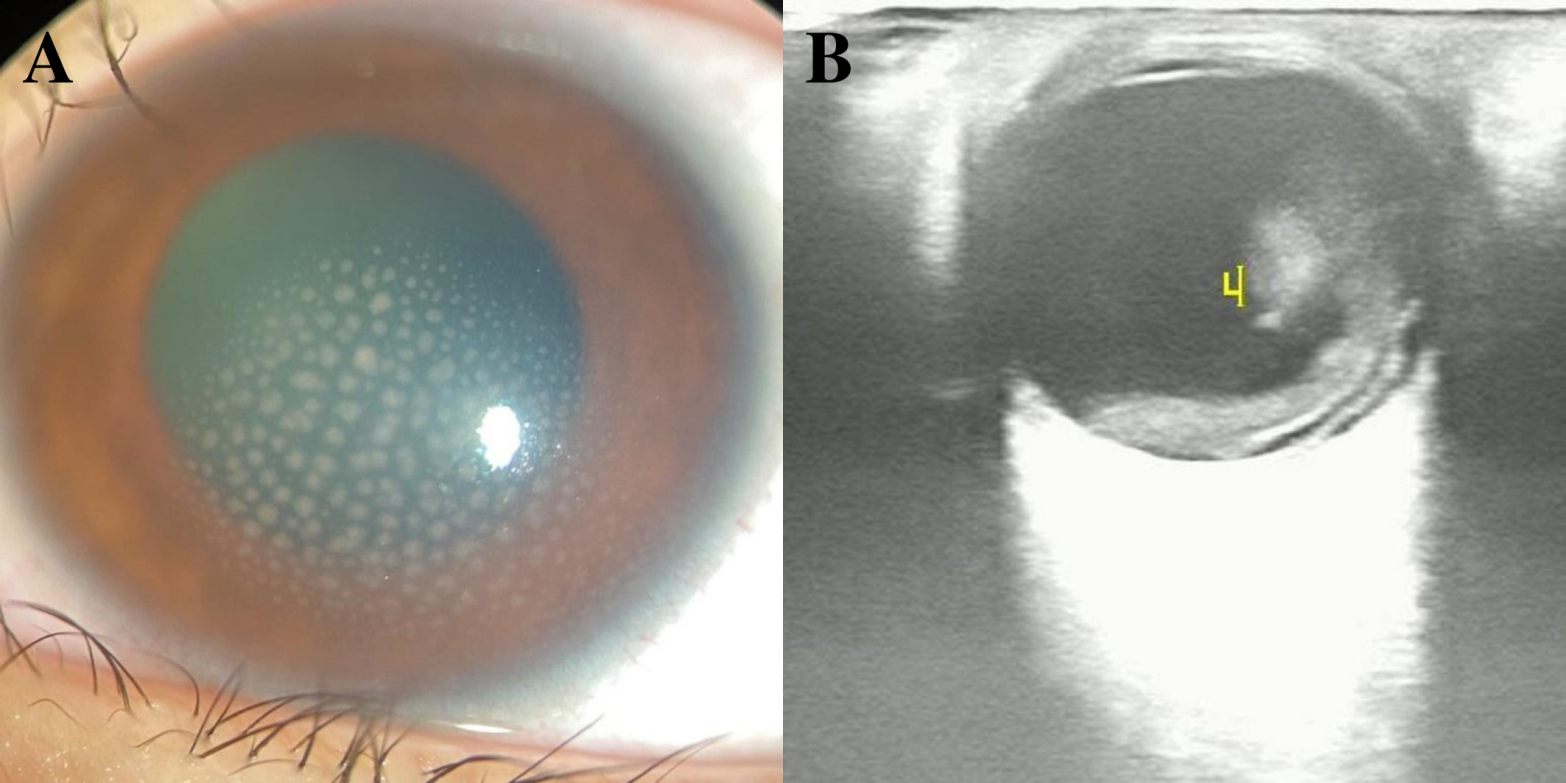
(A) Slit-lamp examination revealed mutton-fat keratic precipitates in the middle and below the corneal endothelium and 3+ cells and 3 + flare in the anterior chamber. (B) Ultrasonography revealed severe vitreous opacity, posterior vitreous detachment in the left eye, and thickening of the posterior wall of the left eyeball.
